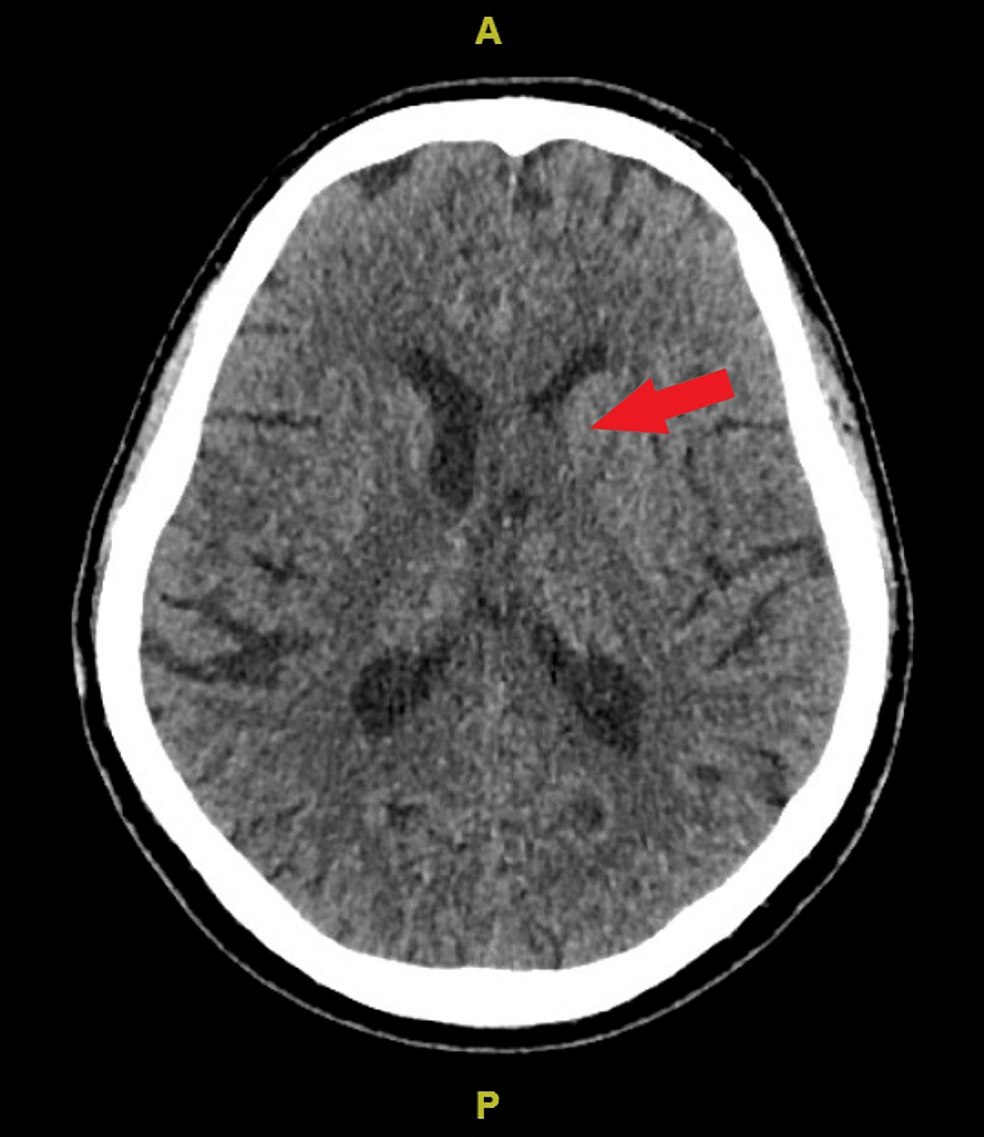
Axial cut of the plain computed tomography image showed a mass compressed on the left lateral ventricle (red arrow).
